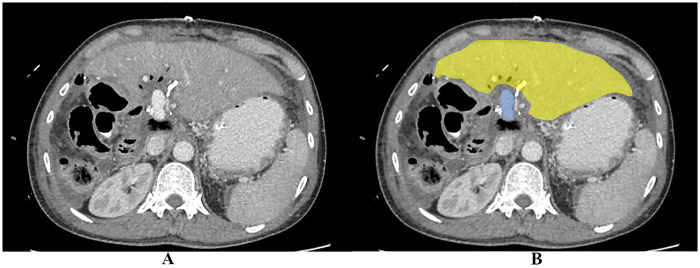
Preoperative CT obtained during the period of bowel ischaemia on POD 4. (A) Unannotated CT demonstrating a patent portal vein without evidence of portal vein thrombosis. (B) Annotated CT with the patent portal vein highlighted in blue and the residual liver remnant in yellow, confirming portal patency in the context of mesenteric venous thrombosis.
